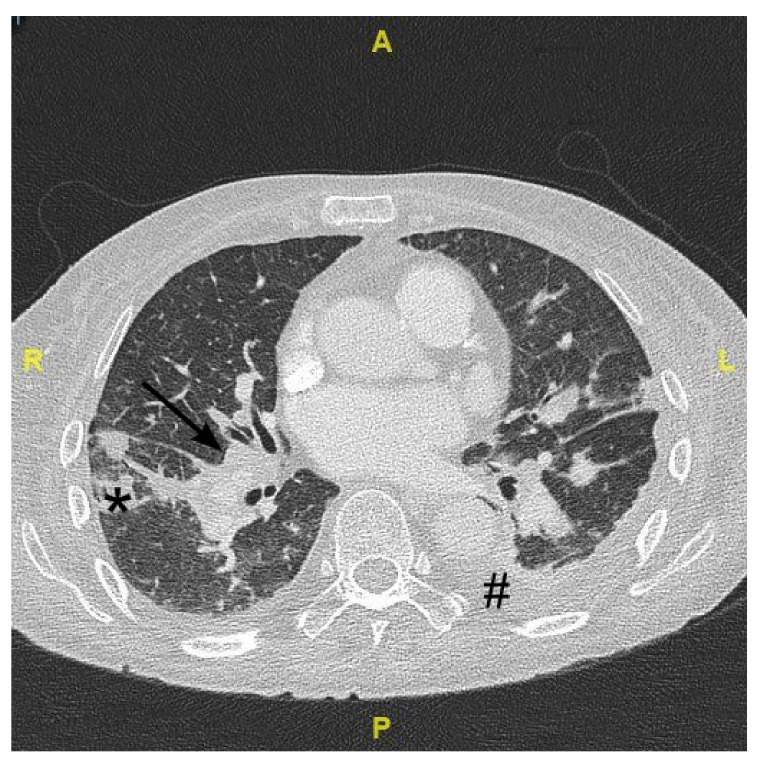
Cropped axial computed tomography image of the thorax on day 36 after admission showing mediastinal and bilateral hilar lymphadenopathy (arrow) as well as focal inflammatory consolidations within the lung parenchyma (star). Additionally, there are small pleural effusion (hash) and accompanying dystelectatic pulmonary areas. Abbreviations: A anterior; P posterior; R right; L left.
